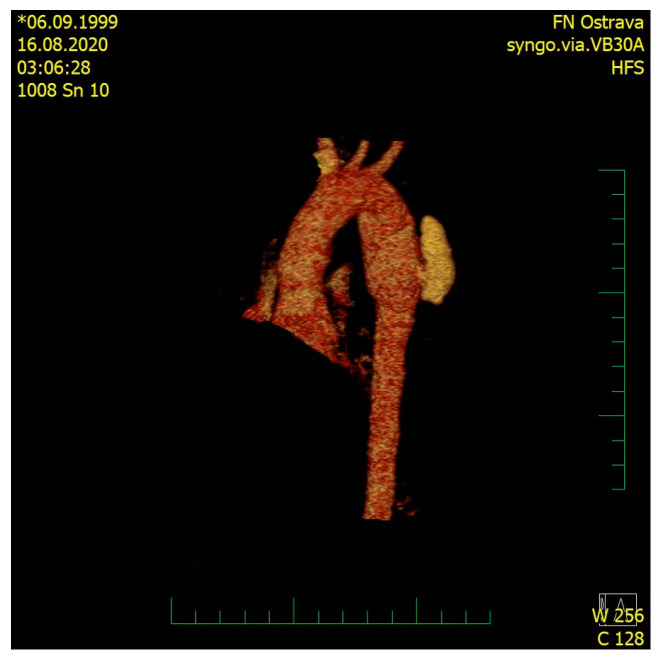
CT 3D reconstruction of the aortic arch with the leak of the contrast agent.
